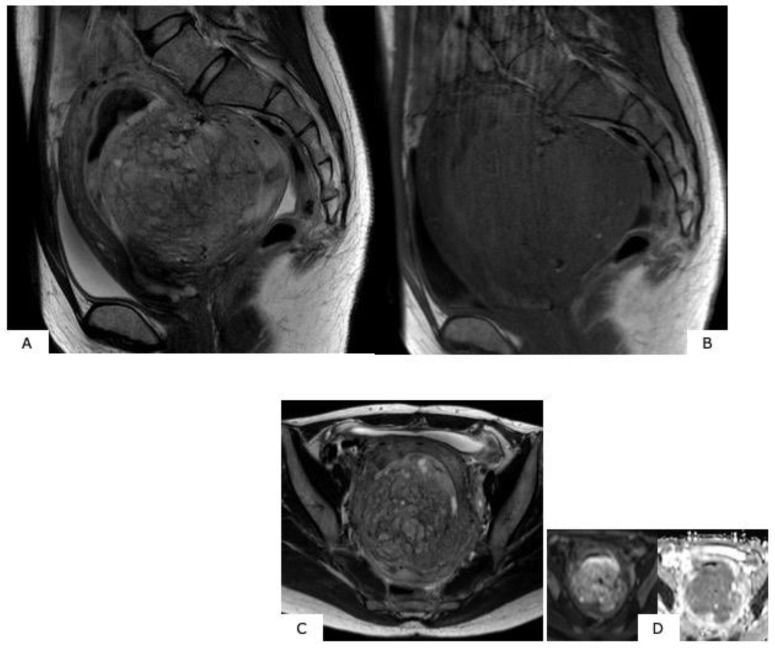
Magnetic resonance images of low-grade endometrial stromal sarcoma. (A): T2-weighted sagittal section, (B): T1-weighted sagittal section, (C): T2-weighted axial section, (D): diffusion-weighted image. Within the uterine posterior wall myometrium, in T2-weighted images, there are relatively high signals, and in T1-weighted images, a mass with ill-defined borders presenting low signals is observed. In (A) and (C), a low-signal band exists in the high-signal mass, presenting a “worm-like” finding that suggests that the low-grade endometrial stromal sarcoma is penetrating the normal myometrium while interposing itself intratumorally. Reduced diffusion is shown in (D).
